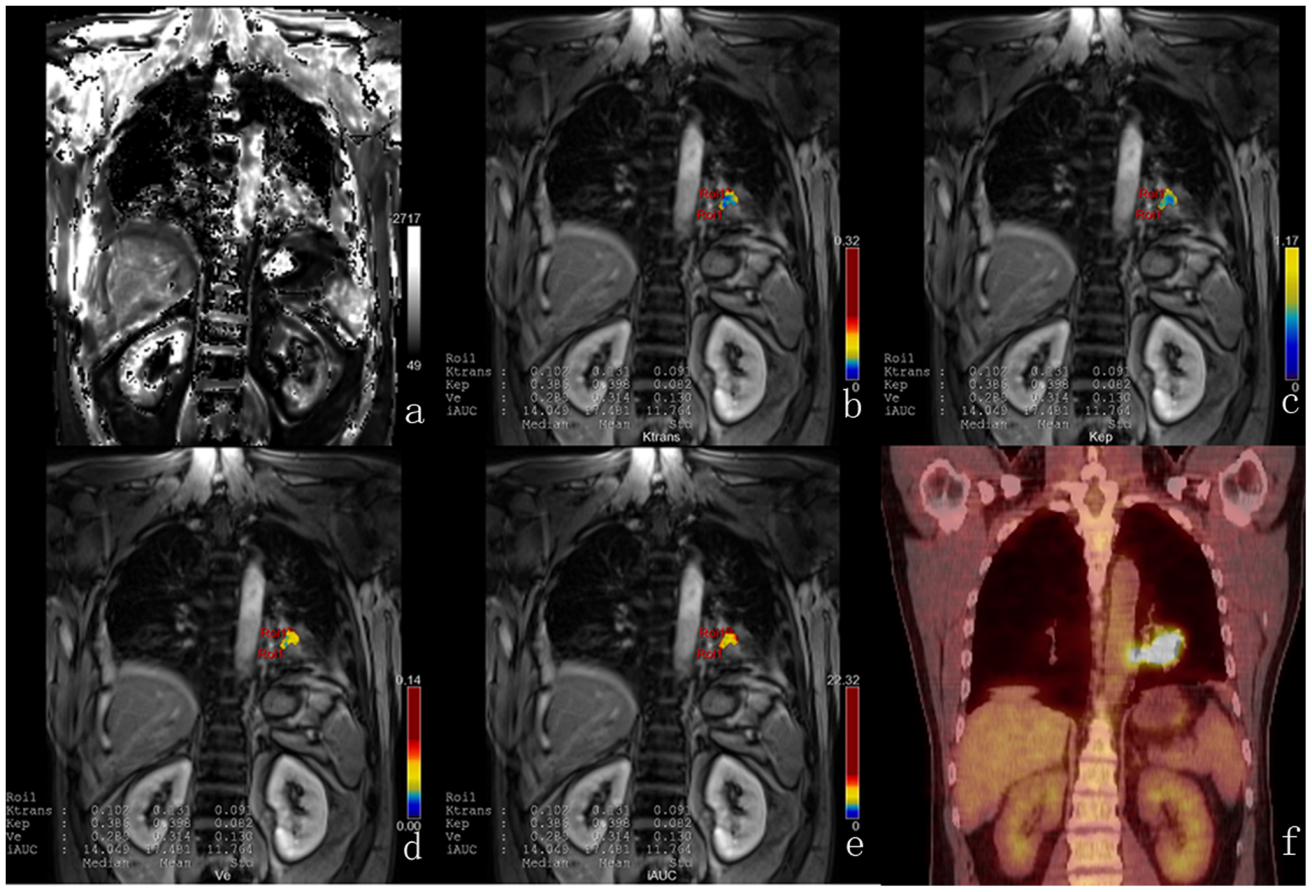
DCE-MRI and PET/CT of a representative case with AC. T1 map (a) is automatically calculated by the precontrast images. Color parametric maps of Ktrans (b), Kep (c), Ve (d) and iAUC (e) based on coronal T1- weighted images of the chest demonstrate the increased tumor microcirculation parameters on the left lower lung. The corresponding PET/CT image (f) shows the avid glucose uptake in the tumor site.
